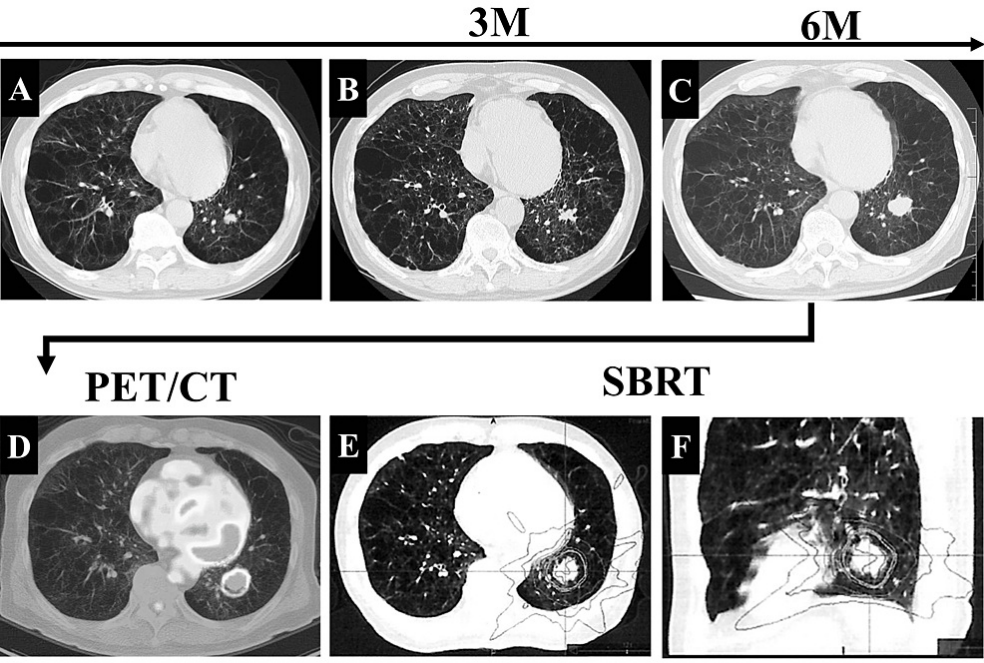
Chest images at the diagnosis and irradiation fields by SBRT. (A) HRCT image of the initially detected nodule. (B, C) Chest CT image three months and six months later. (D) PET/CT scan revealed fluorodeoxyglucose uptake with a maximum standardized value of 20.4. (E, F) Irradiation fields of SBRT. M, months; PET/CT, positron emission tomography/computed tomography; SBRT, stereotactic body radiation therapy; HRCT, high-resolution computed tomography.
